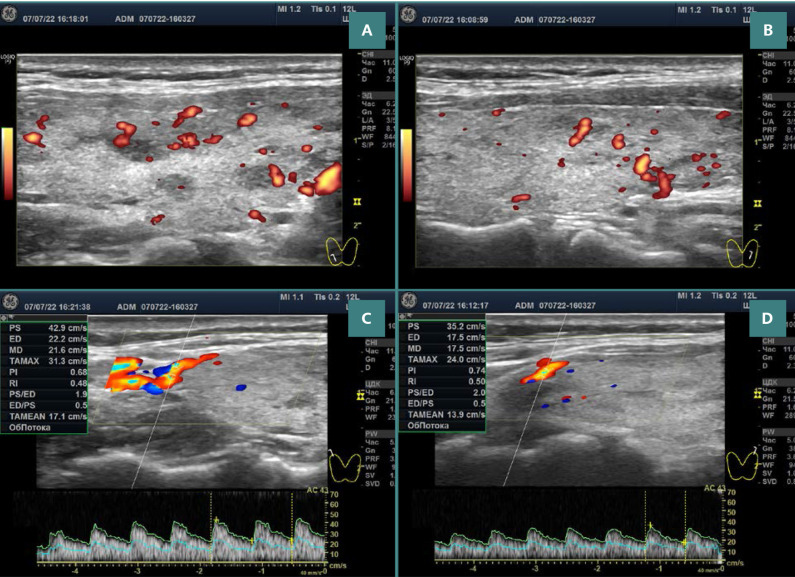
Doppler ultrasonography images of both lobes of the thyroid gland. A,B, Slightly increased blood flow intensity in both lobes (higher intensity is seen in the right lobe). C,D, PSV-STA is 42 cm/s and 35 cm/s in the right and left arteries, respectively (in contrast, the common carotid arteries showed a PSV of 67 cm/s and 42 cm/s in the right and left arteries, respectively, in which the complete arteries were measured at the same level).
